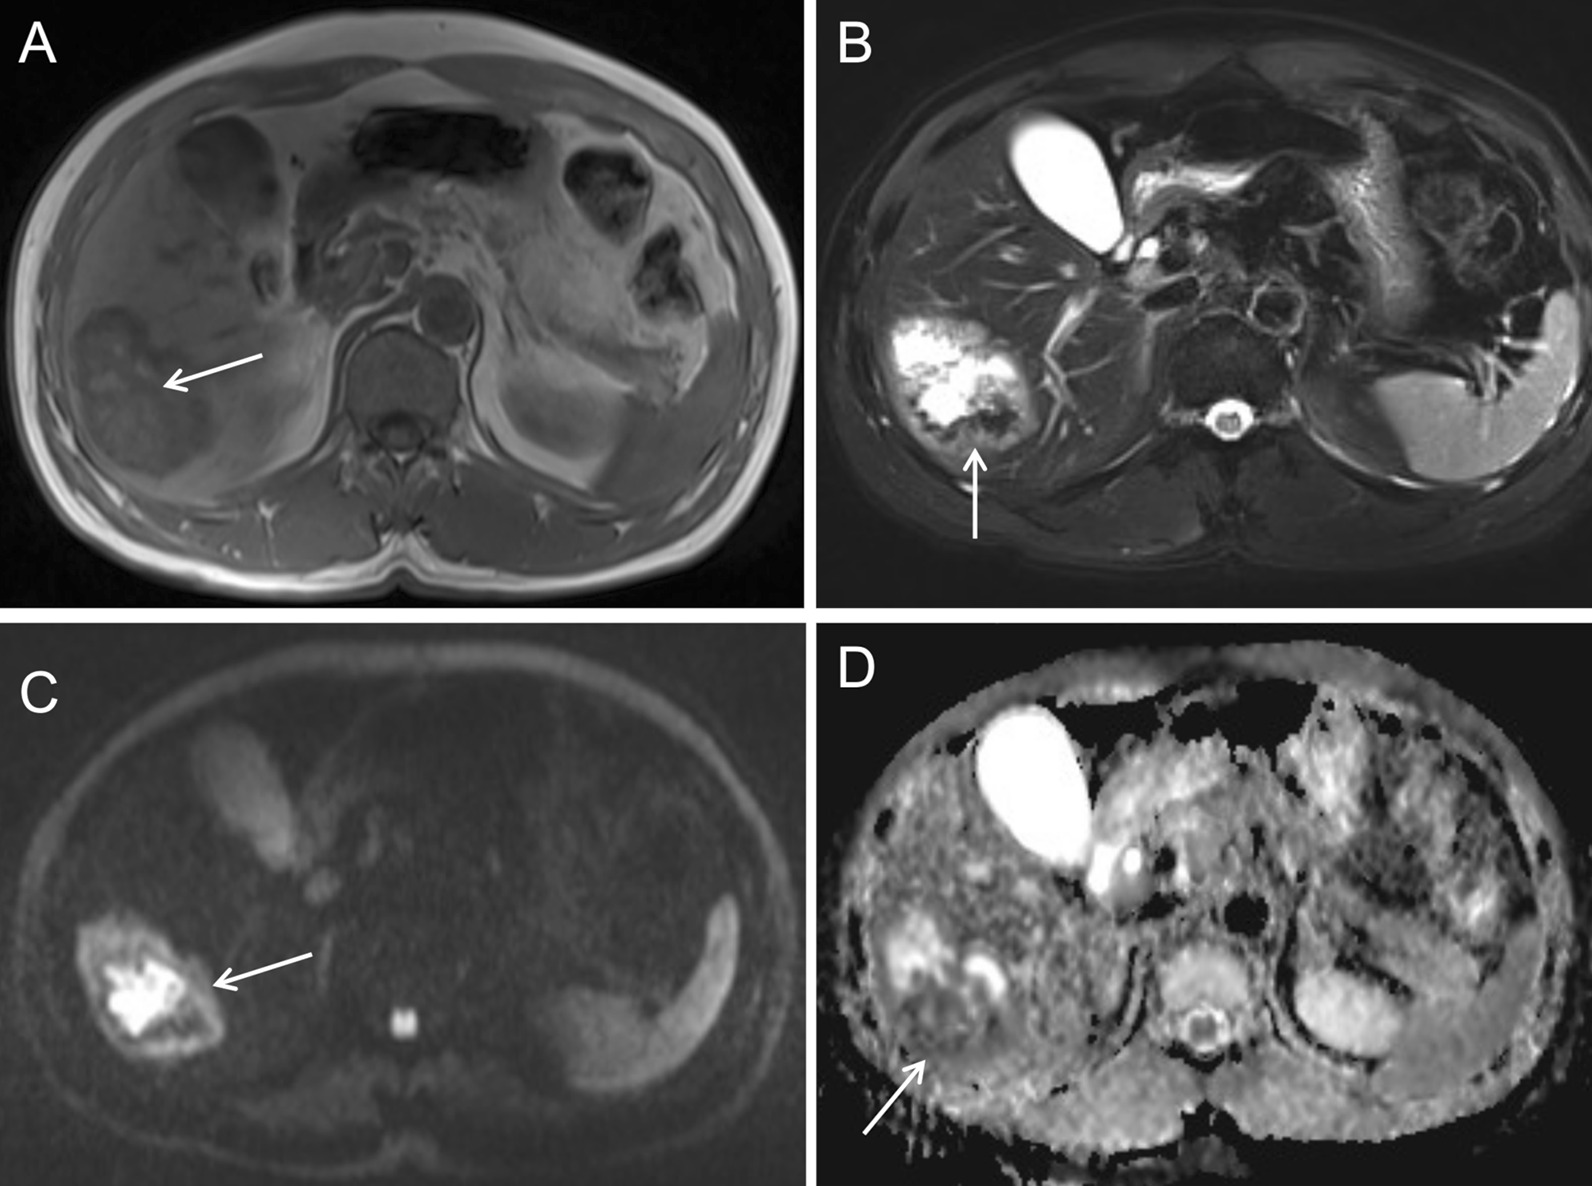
Plain MRI and diffusion-weighted images of case 2
